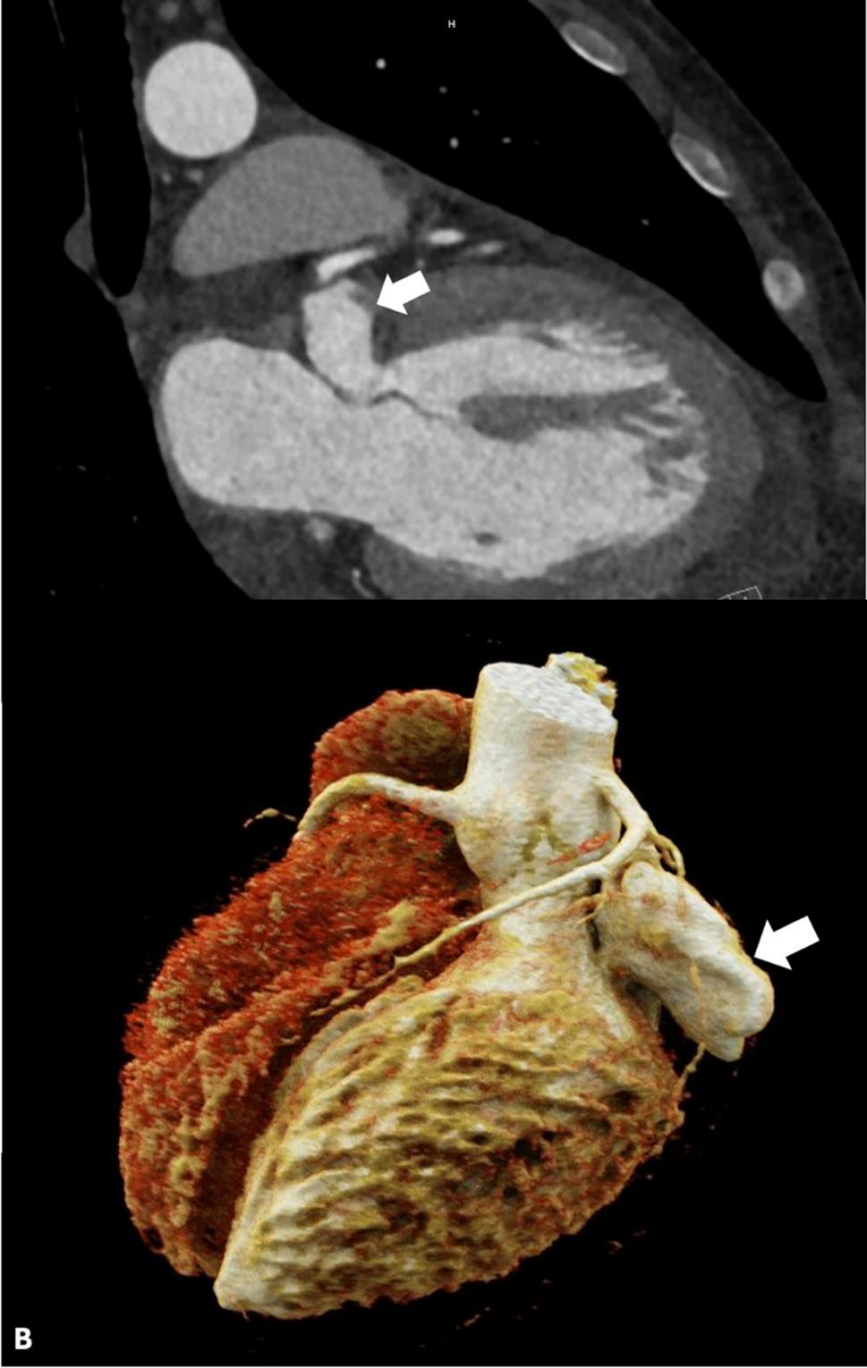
a CT imaging demonstrating the submitral aneurysm at the hinge point of the mitral valve (arrow) b CT 3D reconstruction demonstrating the aneurysm as it appears from the exterior, in close proximity to the left circumflex coronary artery (arrow)
